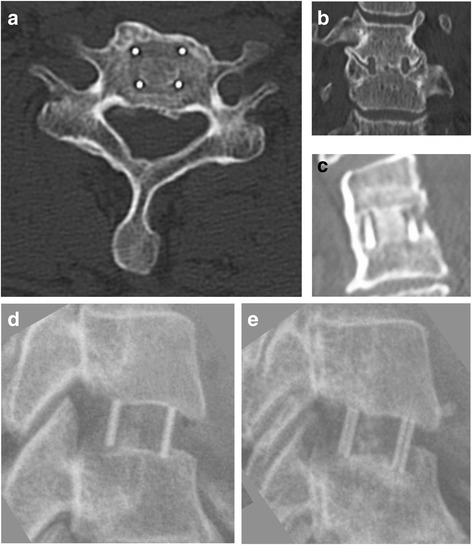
Examples of a good (a–c) and bad (d–e) fusion. The example of a good fusion shows CT-scans from C4/C5 of a 56 year-old man at the 12-month follow-up. a) Axial view. b) Coronal view. c) Sagittal reconstruction. The example of the bad fusion shows X-rays of C5/C6 from a 43 year-old woman. d) Post-Op control. e) At the 6-month follow-up
